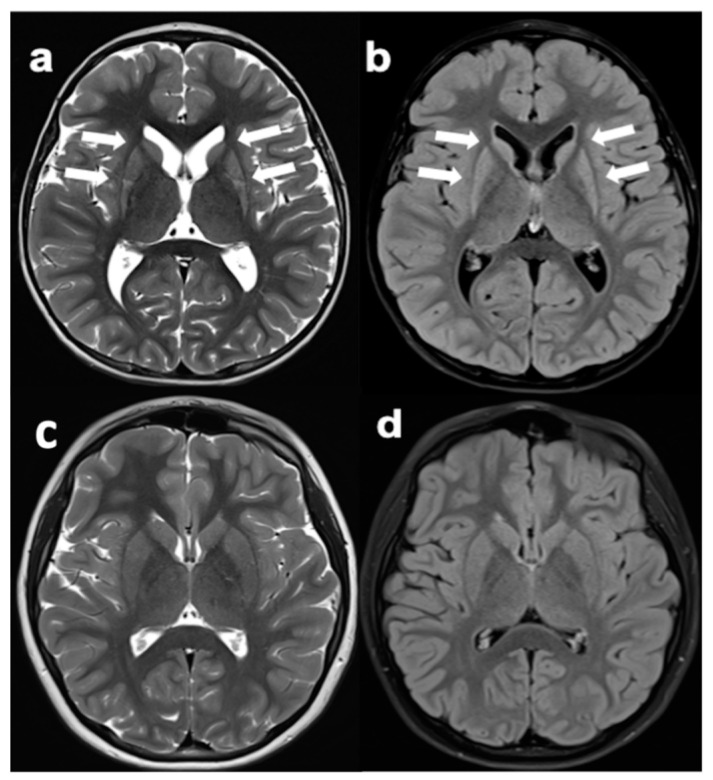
MRI (Magnetic Resonance Imaging) in a HE-PHD (highly expanded- pediatric Huntington disease) patient (Patient 3) and in a subject with suspicious motor manifestations of PHD. (a,b) Patient 3 with a pathological total motor score = 21 performed MRI at the very beginning of PHD (MRI age = 5, age at onset = 3.5). Atrophy and slight signal hyperintensity of the caudate nuclei and putamina (arrows). (c,d) At risk kid with total motor score = 20 and suspicious PHD. Normal appearance of basal ganglia, with no signs of atrophy or signal alterations.
